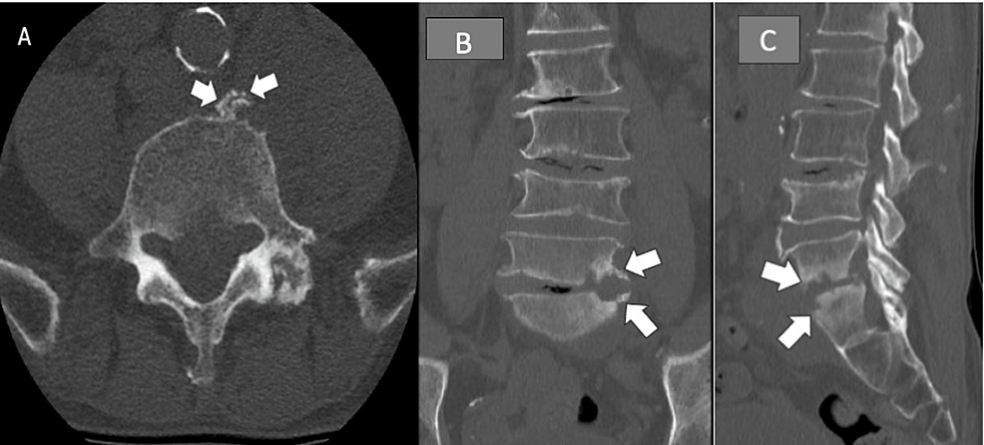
Preoperative multiplanar CT of the lumbar spine without contrast taken on the day of L5-S1 laminectomy for epidural evacuation. Axial (A) image shows the destructive process of the S1 vertebrae with an abscess in the left psoas muscle. Coronal (B) and sagittal (C) images show a deteriorated inferior endplate of L5 and a superior endplate of S1 (arrows).
